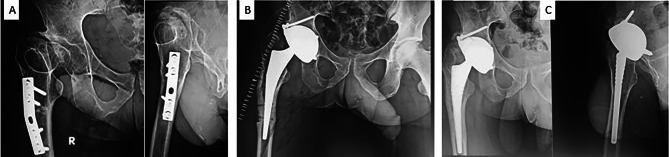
32-year-old male patient had THA for secondary arthritis post-Perthes’ disease. (A) preoperative x-ray that shows the proximal femoral deformity and plate used for varus osteotomy in his childhood (B) immediate post-operative x-ray with Libra cementless stem inserted through the site of previous deformity and screw holes evident around distal part of the stem. (C) 4 years post-operative with stem integration and filling of bone defects
